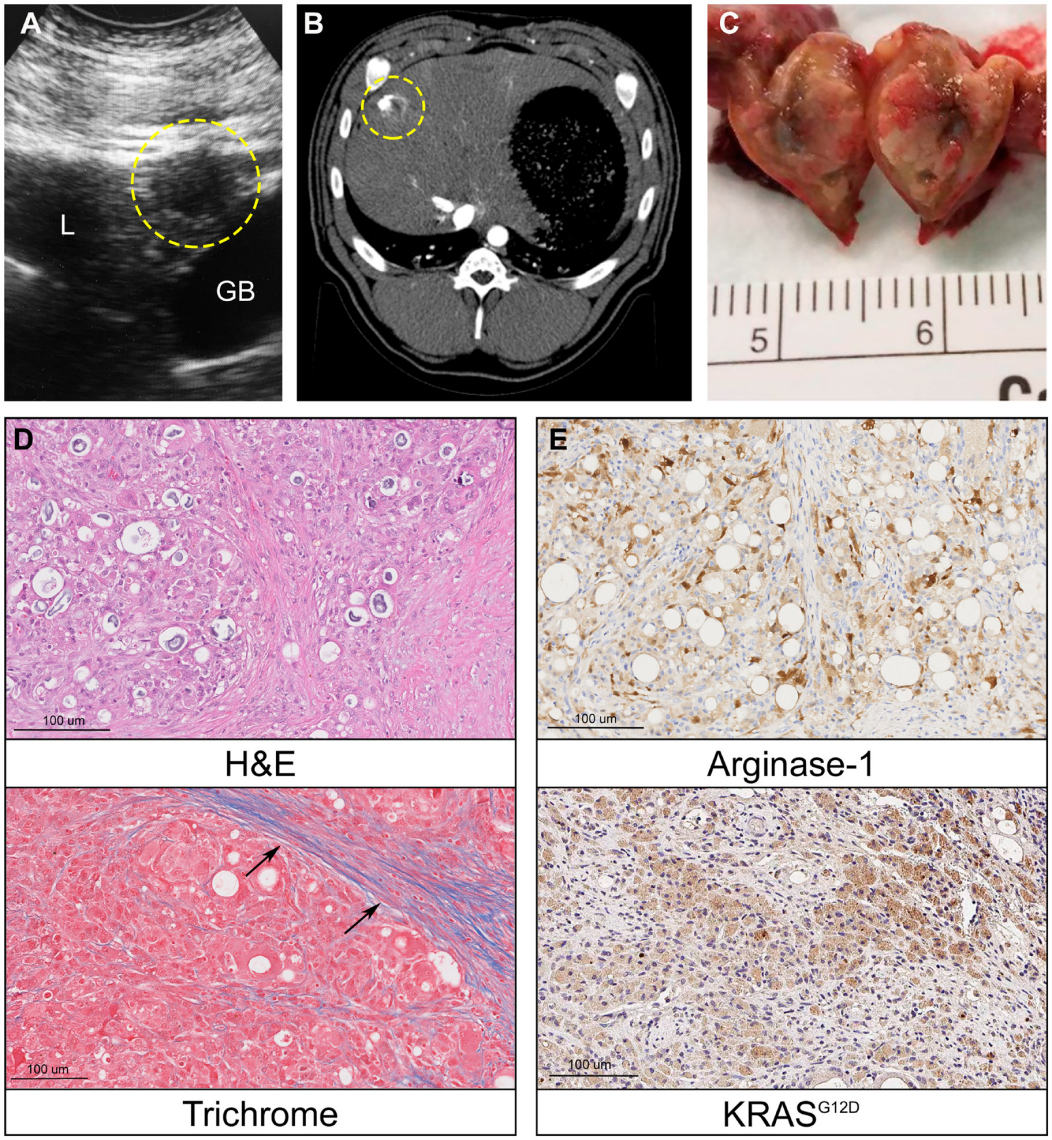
Oncopig intrahepatic HCC tumor formation. (A) Liver ultrasound depicting a hypoechoic 1 cm round intrahepatic HCC tumor (circled, L = liver, GB = gallbladder). (B) Contrast enhanced liver CT depicts same HCC tumor (circled). (C) Photograph of transected intrahepatic HCC tumor. (D) H & E (20×) of Oncopig intrahepatic HCC tumor reveals architectural distortion characterized by expansion of liver cords, nuclear pleomorphism, anisonucleosis, and nodular fibrosis. Masson’s trichrome of adjacent non-tumorous liver demonstrates dense collagen bands (arrows) consistent with METAVIR grade 2-3 fibrosis. (E) Arginase-1 IHC (20×) shows patchy arginase-1 expression (brown) consistent with hepatocellular differentiation. KRAS^G12D^ IHC (20×) confirms KRAS^G12D^ expression (brown) consistent with malignancy.
